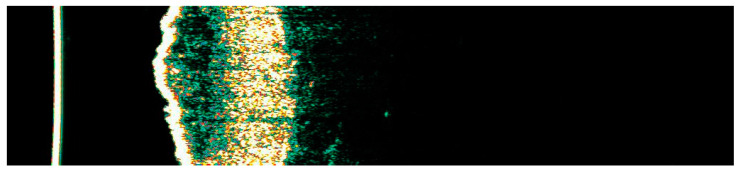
Ultrasonographic image of BCC on the nose.
